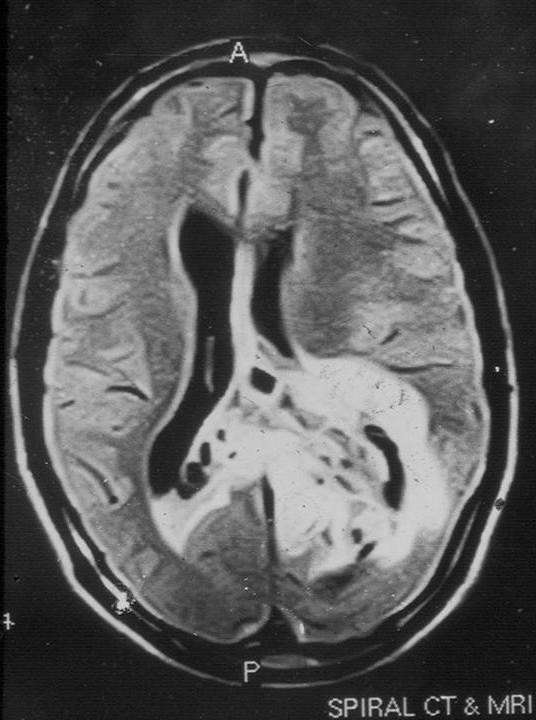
Non-contrast magnetic resonance imaging showing hyper-intense lesion involving the left temporal and parieto-occipital regions. The tumor is crossing the midline to the right parietal region.
